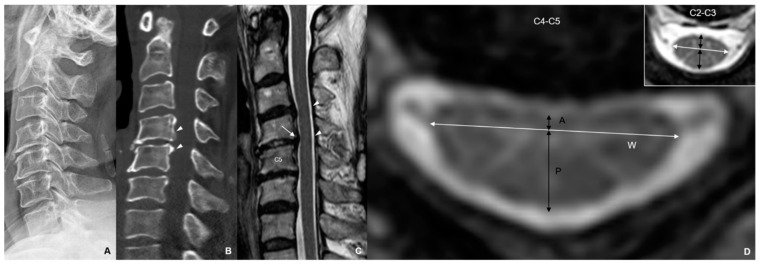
Radiographic images of a 56-year-old female patient with hand clumsiness and gait disturbance. Her total Japapanese Orthopadic Association (JOA) score was 13 (UEF-2, LEF-3, sensory-5, and bladder function-3). (A) Cervical lateral X-ray with normal alignment (at neutral position); (B) segmental ossification of posterior longitudinal ligament was shown at C4 and C5; (C) anterior compression of spinal cord was shown at C4−C5 segment (white arrow), but sufficient space behind the spinal cord was observed (arrow heads). (D) At C4−C5 segment, anterior (A) and posterior (P) lengths of the spinal cord were 0.85 and 4.84 mm, respectively, and anteroposterior (A-P)/width (W) ratio was 0.38. Anterior and posterior lengths of the spinal cord at C2−C3 were 2.56 and 4.84 mm, respectively, and AP/W ratio was 0.59. At 2 years after laminoplasty, her total JOA score improved from 13 to 16 (UEF-4, LEF-3, sensory-6, and bladder function-3) (UEF: upper extremity function); LEF: lower extremity function).
